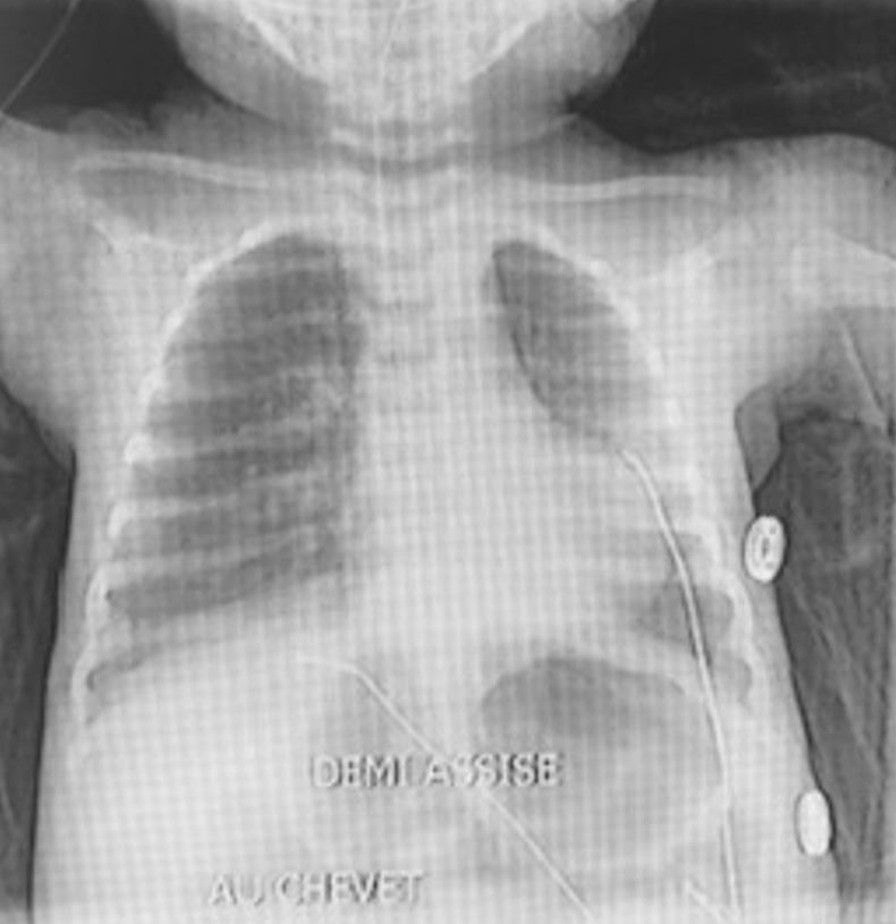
Chest X-ray upon admission to the pediatric ward
